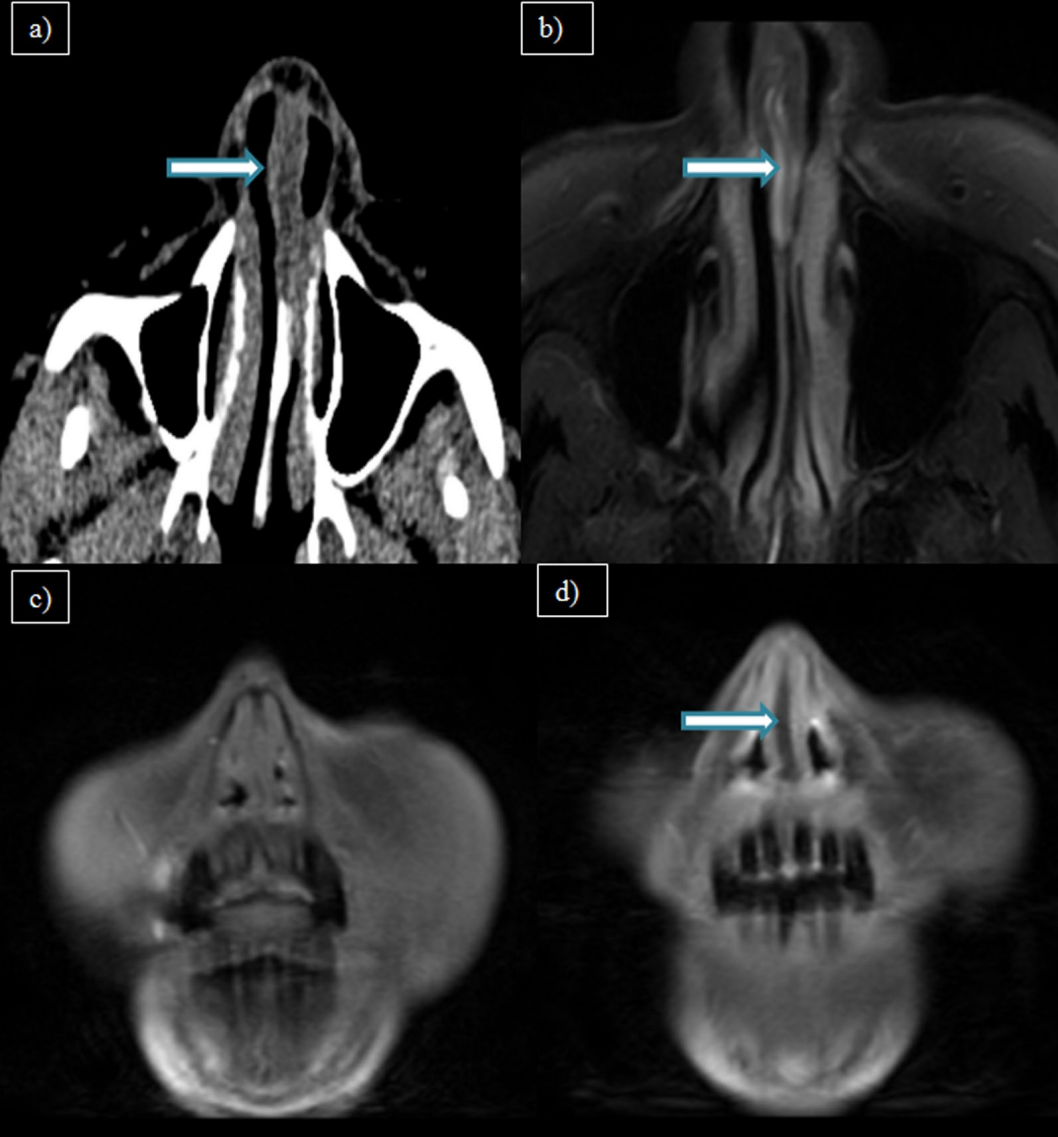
a Axial CT paranasal sinus (soft tissue window) of a 68-year-old female patient with complaints of nasal obstruction showing thickened nasal septum with hypodense collection (arrow). b MRI axial T2W-FS, c coronal pre-contrast T1W-FS and d coronal post-contrast T1W-FS images of same patient showing peripherally enhancing collection in the cartilaginous part of nasal septum(arrow)–nasal septal abscess
